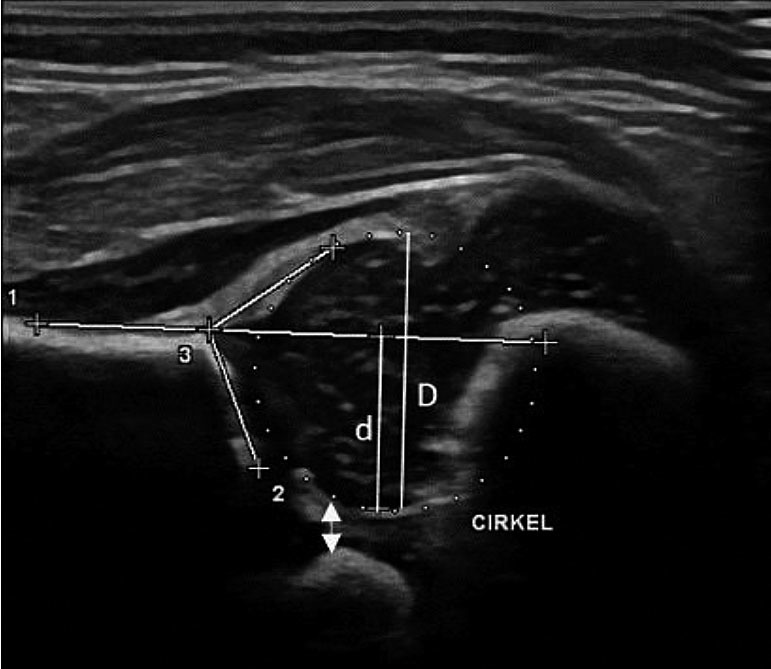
Ultrasound image of normal hip at 4 weeks. All anatomical landmarks present. The horizontal line extending from the iliac wing crosses the perpendicular femoral head diameter and defines the upper limit for d which is used to calculate BRP = d/D. The PFD is marked here by the double-headed arrow.
